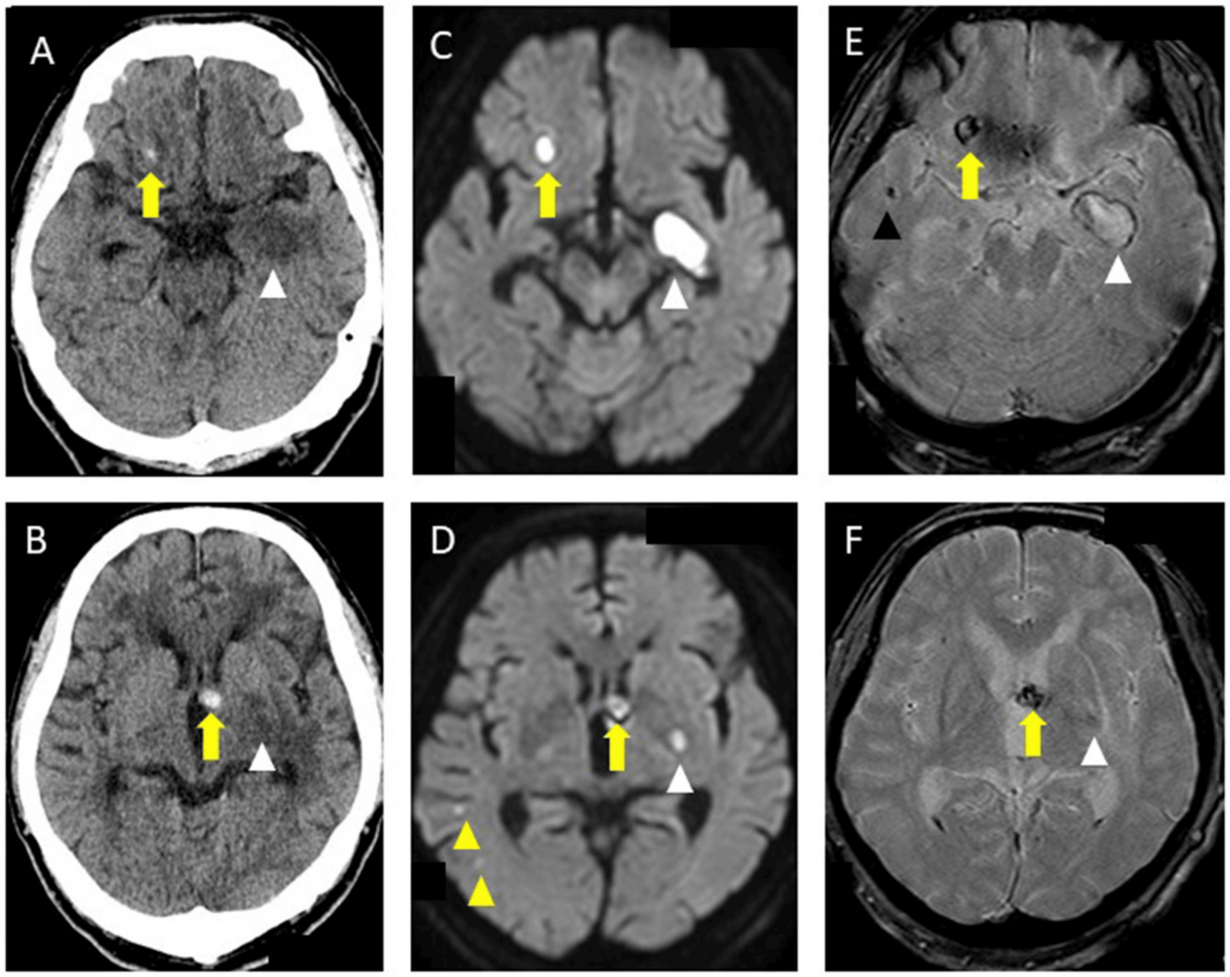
Brain computed tomography (CT), magnetic resonance imaging (MRI) diffusion-weighted imaging (DWI), and T2* imaging on day 122 A, B: Brain CT revealed acute cerebral hemorrhage in the right frontal subcortex and left internal capsule genu (yellow arrow), and new low-density area in the left temporal lobe and basal ganglia (white triangle). C, D: Brain MRI-DWI demonstrated new small high signals in the right left temporal sub-cortex of new small infarctions (yellow arrow), new high signals in the right frontal subcortex and left internal capsule genu of acute cerebral hemorrhage (yellow arrow), and new high signals in the left temporal lobe and left basal ganglia of sub acute intracerebral hemorrhage (white triangle). E, F: T2* imaging revealed new right temporal microbleeds (black triangle), annular low intensity in the right frontal subcortex and left internal capsule genu of acute cerebral hemorrhage (yellow arrow), and annular low intensity in the left temporal lobe and left basal ganglia of subacute cerebral hemorrhage (white triangle).
